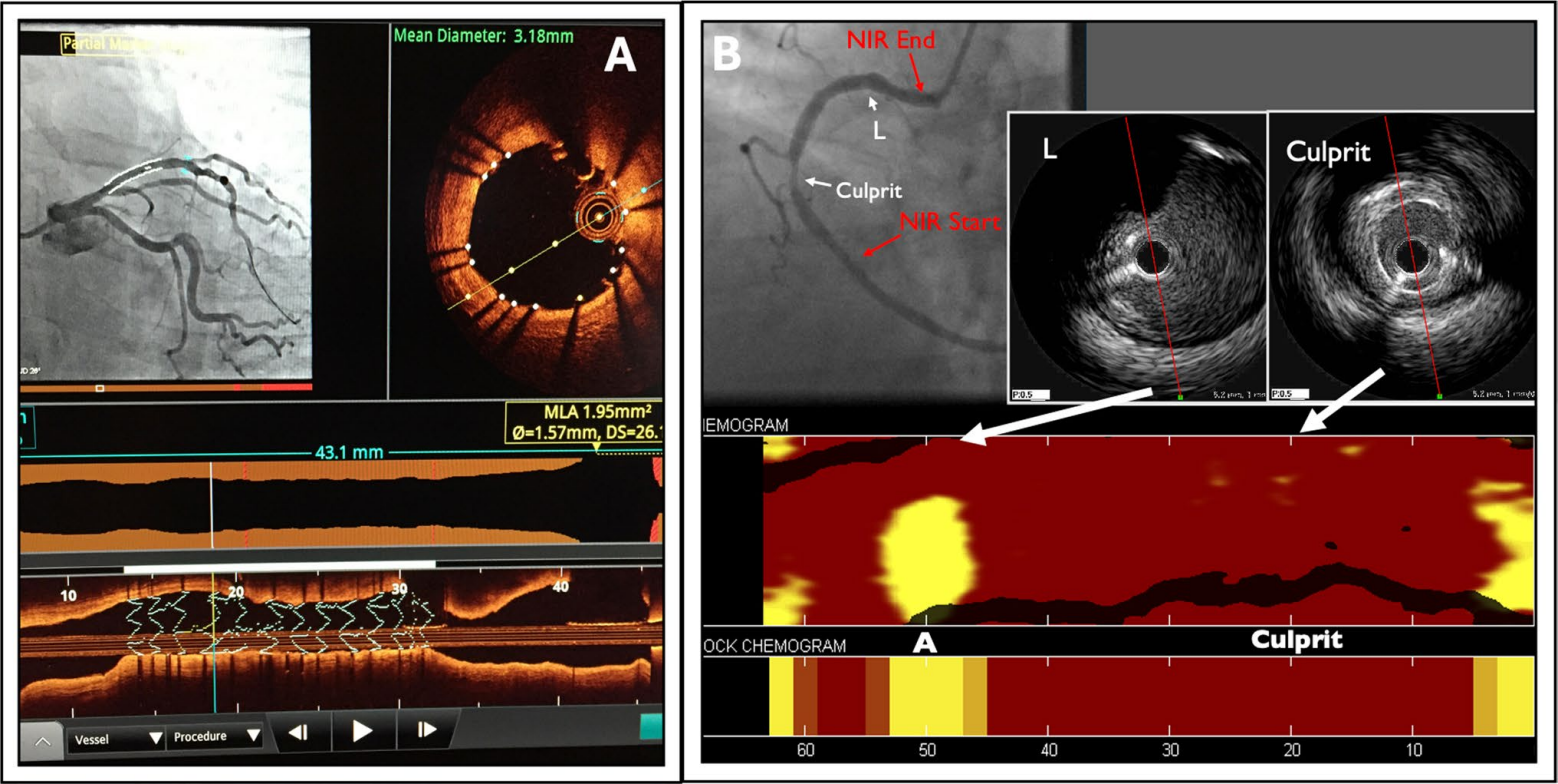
A. OCT display showing co-registration with the angiogram with its corresponding cross-sectional OCT frame at the point of the cursor (top). The stent struts (*white dots*) are well apposed to the vessel wall. Bottom part of the display shows longitudinal reconstruction of the artery with the stent in place and the luminogram that allows for precise measurements of lumen diameter, area, and lesion length. B. Right coronary angiogram still-frame with its corresponding chemogram obtained with NIRS-IVUS pullback. The culprit stenosis reveals significant lumen narrowing by IVUS but low probability of lipid by NIRS. A more proximal non-culprit area (L) reveals a high probability of lipid in a non-obstructive plaque by IVUS and angiography. Key: OCT: Optical coherence tomography. NIRS: Near-infrared spectroscopy. IVUS: Intravascular ultrasound
